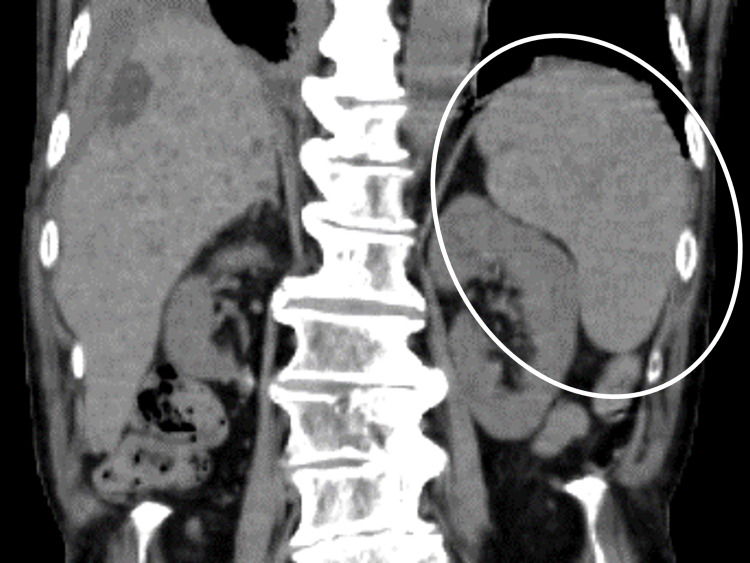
Abdominal CT showing splenomegaly (white circle). CT: computed tomography.
